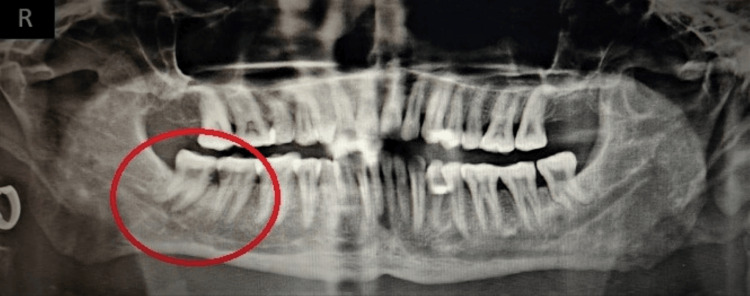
Orthopantomogram showing proximal caries in the 47 and 48 Image credit: Prasanna R. Sonar
